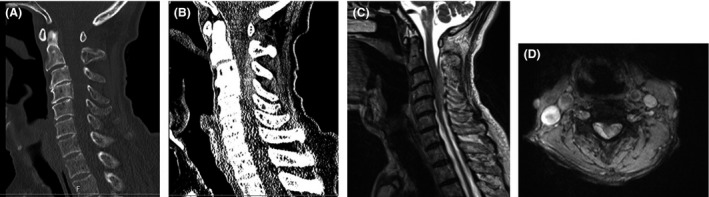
Imaging findings from case 2, a 90‐year‐old Japanese man suddenly developed dysstasia without back or neck pain. A, B, Bone‐specific computed tomography reveals no definite findings, although a high‐density soft tissue mass is visible in the spinal canal at C2–C5. C, D, Sagittal and axial T2‐weighted magnetic resonance imaging reveals a spontaneous spinal subdural hematoma on the dorsal side of the spinal cord at C3–C5.
